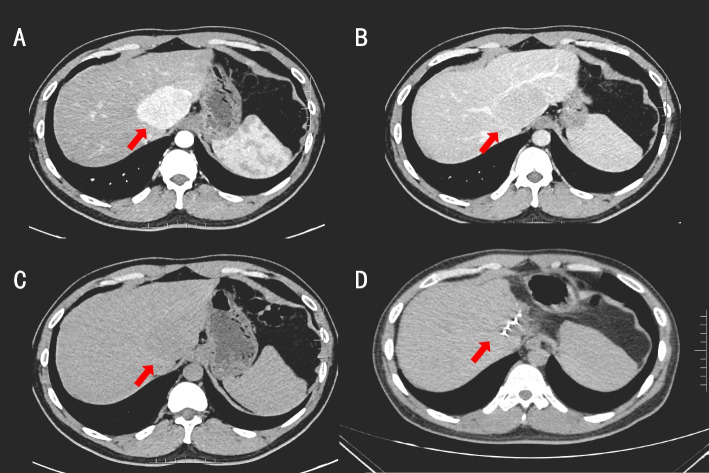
A Sagittal-plane enhanced CT arterial phase images (arrow indicates the lesion in segment). B Sagittal-plane contrast-enhanced CT venographic phase image (arrow indicates the lesion in segment). C Sagittal-plane contrast-enhanced CT delayed phase images (arrow indicates the lesion in segment). D Postoperative CT images of the patient (arrow points to the area of the lesion following surgery)
